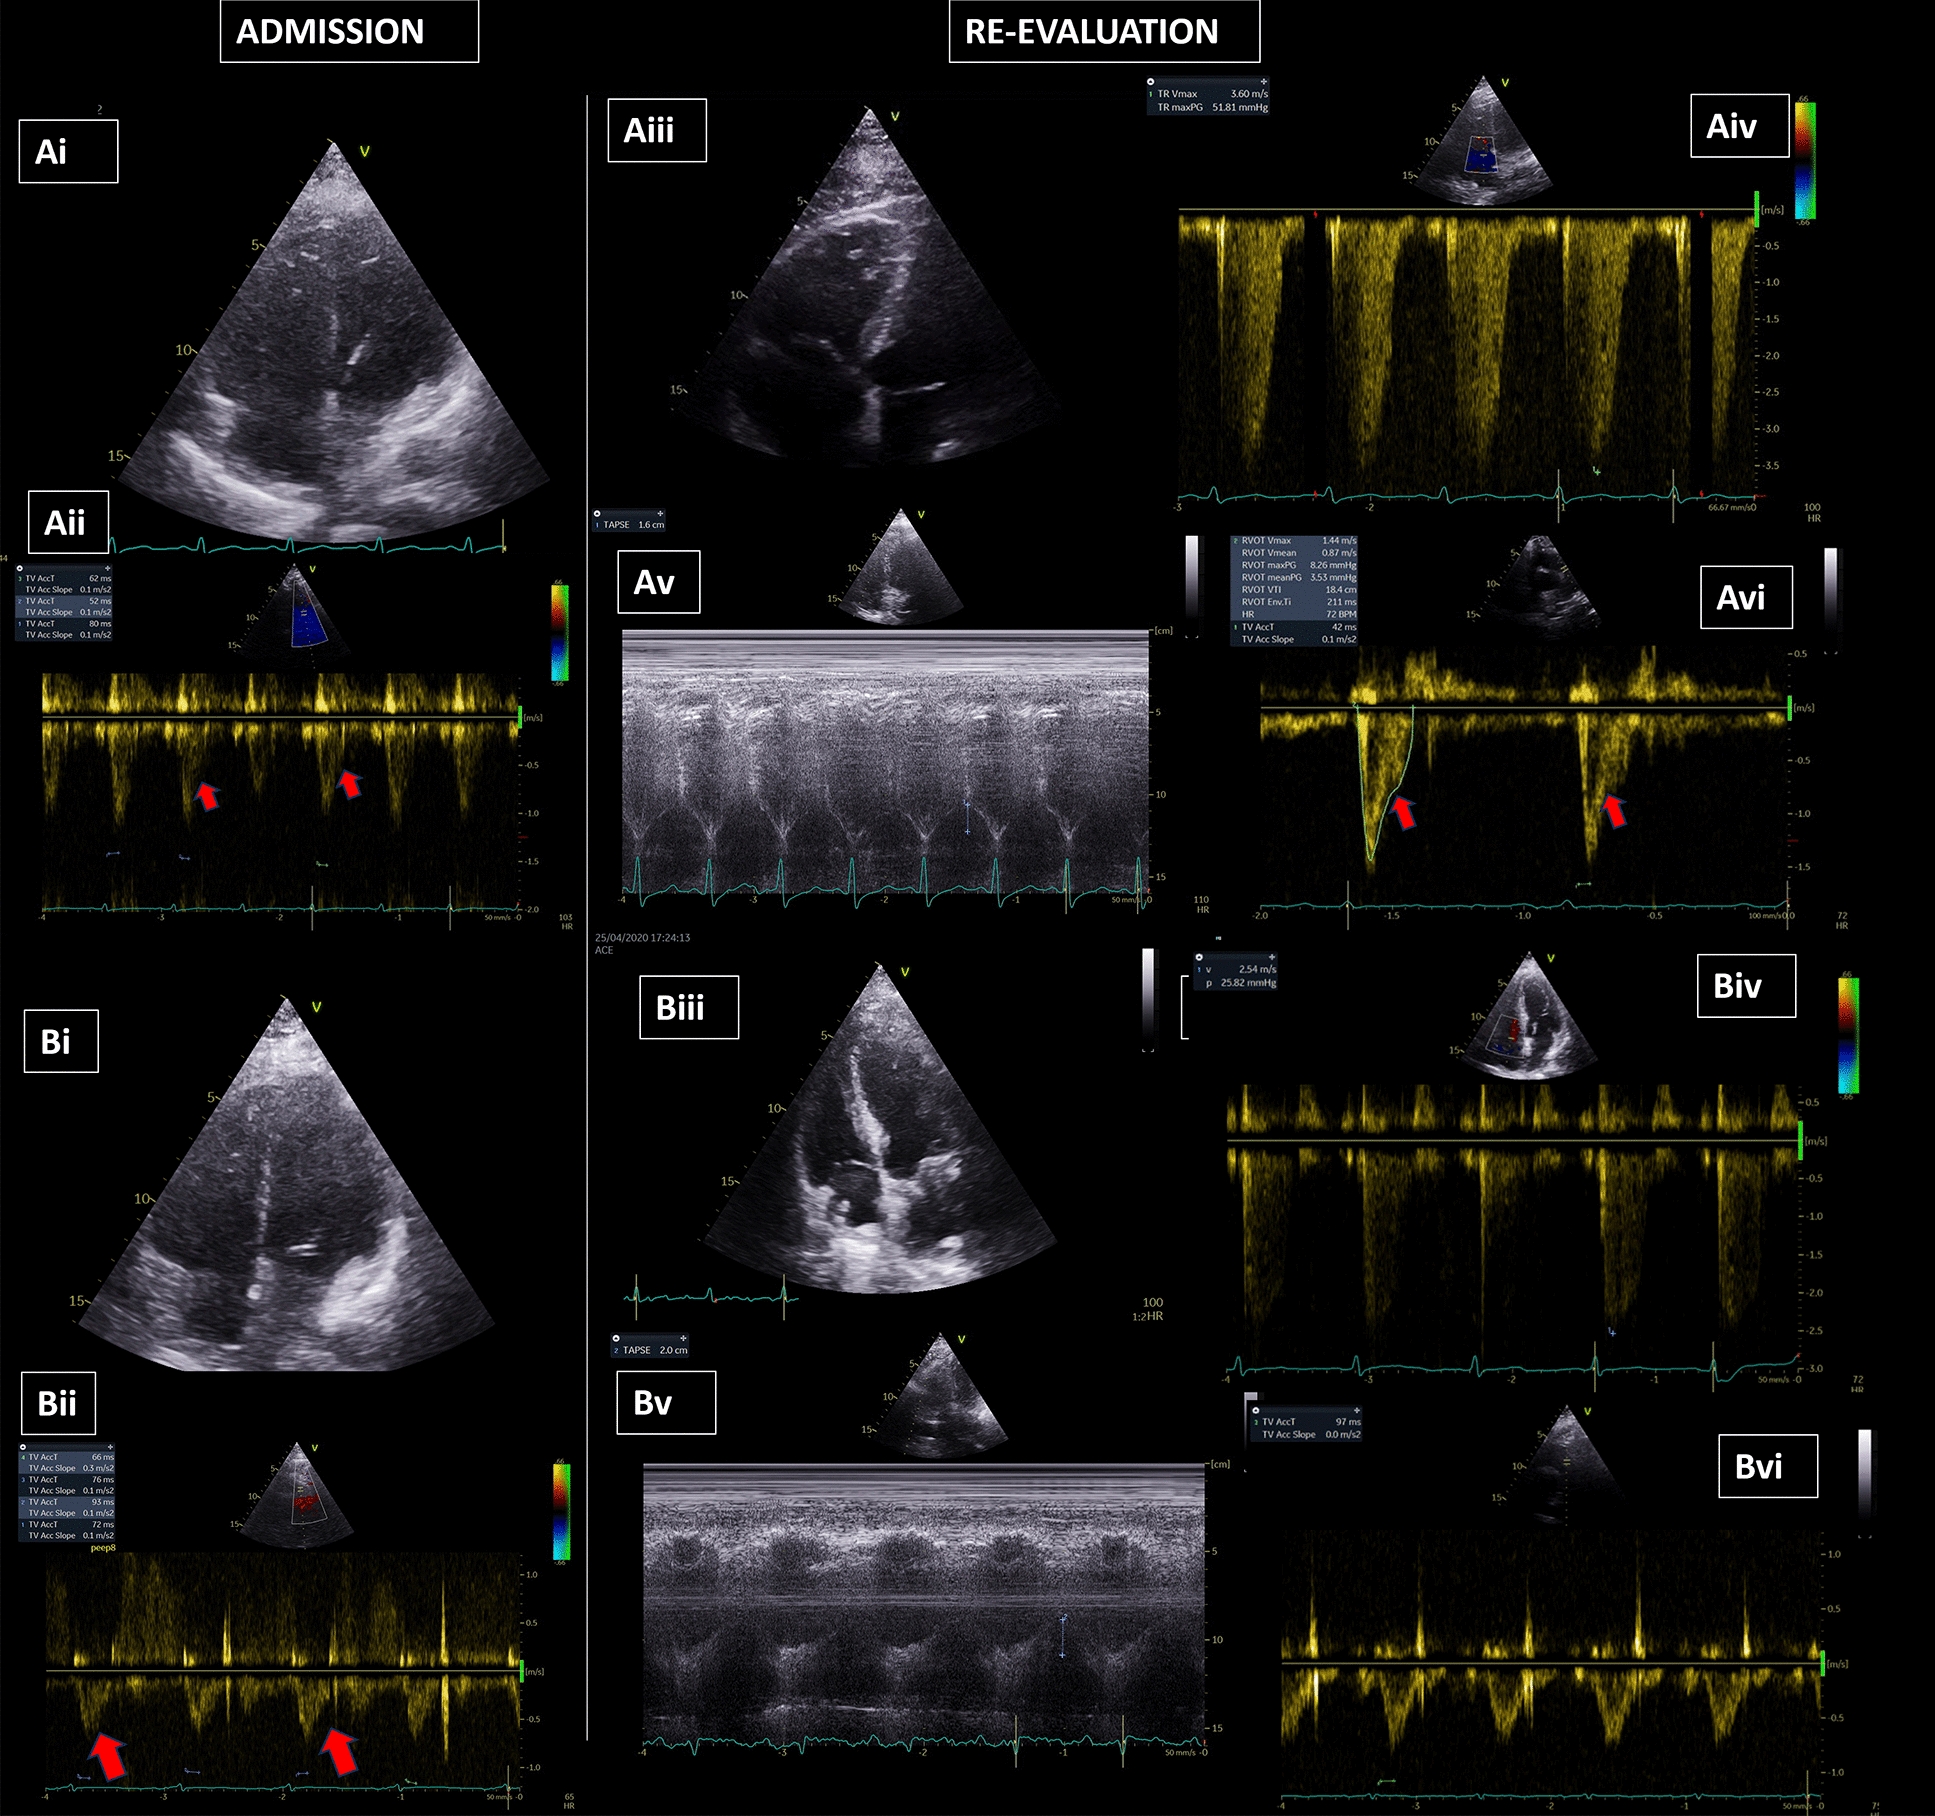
Echocardiographic findings in patients with COVID-19 ARDS. A Non-survivor patient. (Αi) non-survivor with RV dilation on ICU admission; (Aii) PAcT: 64.6 ms Red arrows indicate the early systolic notch in the ascending part of the RVOT envelope. Please not the triangular spahe of the RVOT envelope indicating increased PVRs. (Aiii) RV dilatation upon re-evaluation; (Aiv) TRV: 3.6 m/sec ≥ PASP = 51.81 mmHg + (CVP: 13 mmHg) = 64.25 mmHg (Av) TAPSE: 1.6 cm (re-evaluation); (Avi) PAcT: 42 ms Red arrows indicate the early systolic notch in the ascending part of the RVOT envelope. Please not the triangular spahe of the RVOT envelope indicating increased PVRs. B Survivor patient. (Bi) survivor with RV dilatation upon ICU admission; (Bii) PAcT: 76.75 ms Red arrows indicate the early systolic notch in the ascending part of the RVOT envelope. Please not the triangular spahe of the RVOT envelope indicating increased PVRs, (Biii) normal size of the RV upon re-evaluation; (Biv) TRV: 2.54 m/sec ≥ PASP = 25.82 mmHg + (CVP: 8 mmHg) = 33.82 mmHg (Bv) TAPSE: 2 cm; (Bvi) PAcT: 95 ms, Please not the normal parabolic shape of the RVOT envelope in indicating normal PVRs. *ARDS* acute respiratory distress syndrome, *CVP* central venous pressure, *COVID-19* coronavirus disease 2019, *PASP* pulmonary artery systolic pressure, *PVR* pulmonary vascular resistance, *PAcT* pulmonary acceleration time, *RV* right ventricle, *TAPSE* tricuspid annular plane systolic excursion, *TRV* tricuspid regurgitation velocity
